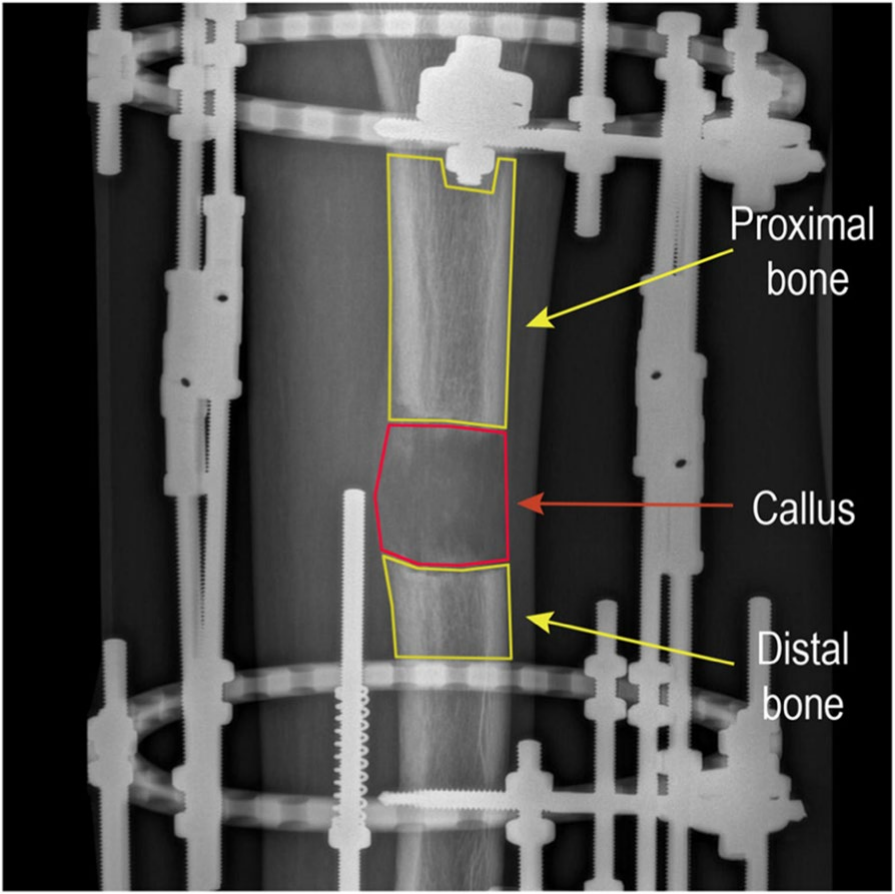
The assessment of PVR based on radiographic
